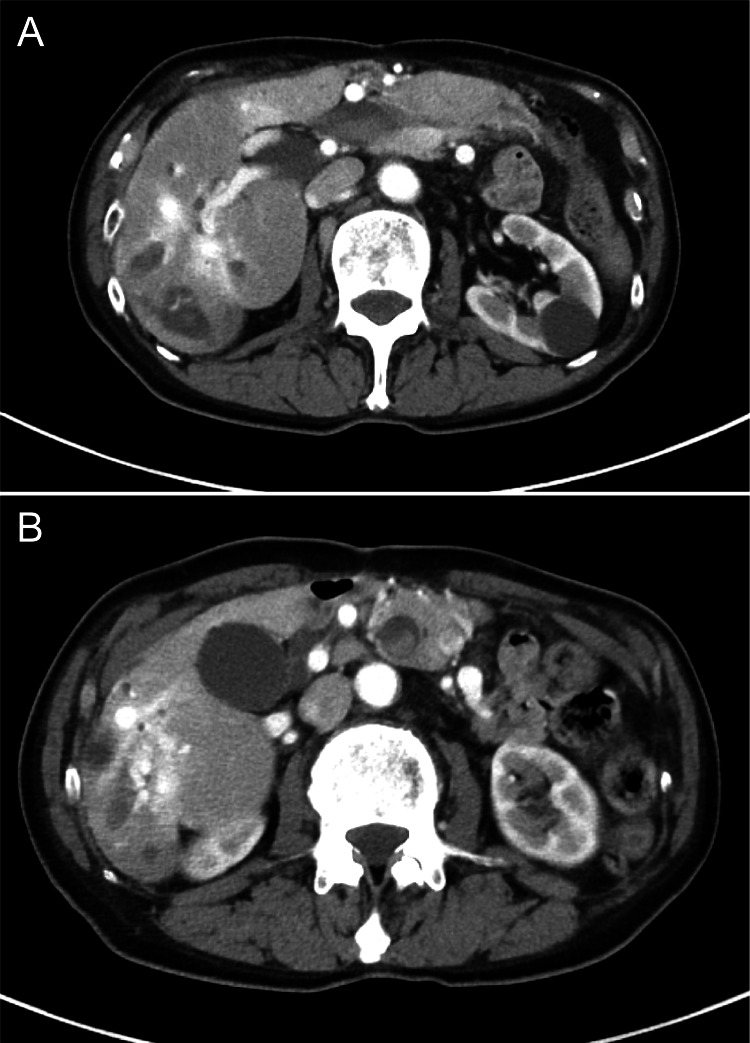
Contrast-enhanced computed tomography (CT) images showing hemobilia and hematoma (a) CT scan of the S6 segment of the liver, indicating suspected bleeding within the bile ducts, characterized by high-density areas suggesting active hemorrhage; (b) Detailed image of the common bile duct showing the presence of a hematoma visualized as an encapsulated high-density region within the ductal structure
